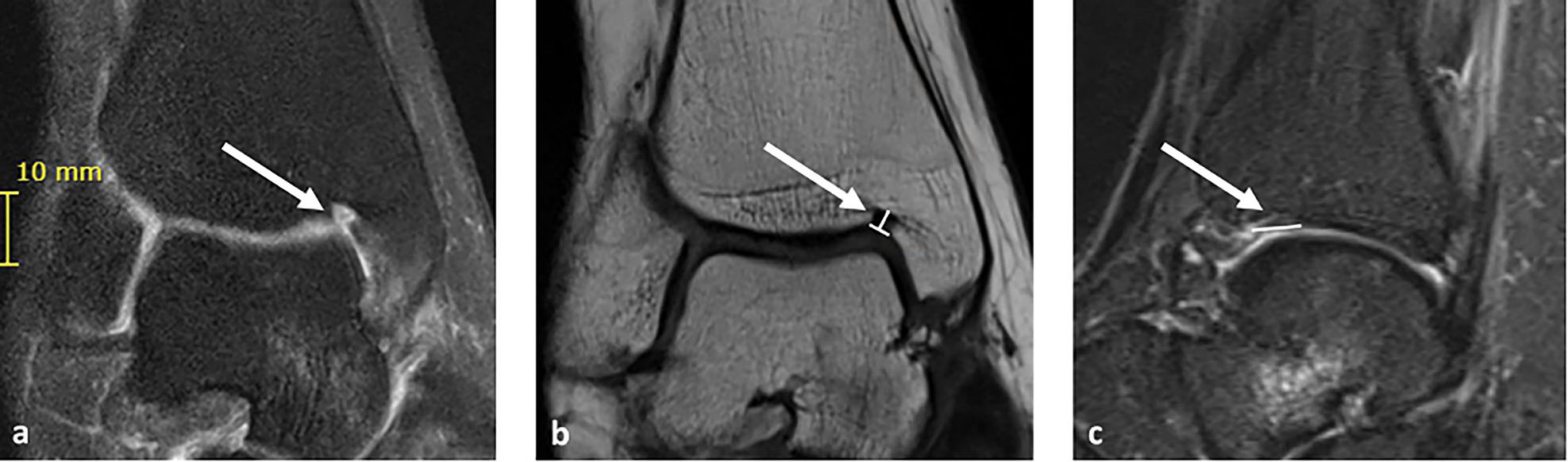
MRI of the right ankle in a 15-year-old female participant demonstrating a type 1 NOH (white arrows). a Coronal proton density-weighted turbo spin echo with fat suppression, (b) coronal T1-weighted turbo spin echo (TSE) with calipers measuring height and width, and (c) sagittal turbo inversion recovery magnitude (TIRM) sequence with fat suppression and contrast with calipers measuring depth. The NOH dimensions are 3 mm (height), 3 mm (width), and 6 mm (depth)
